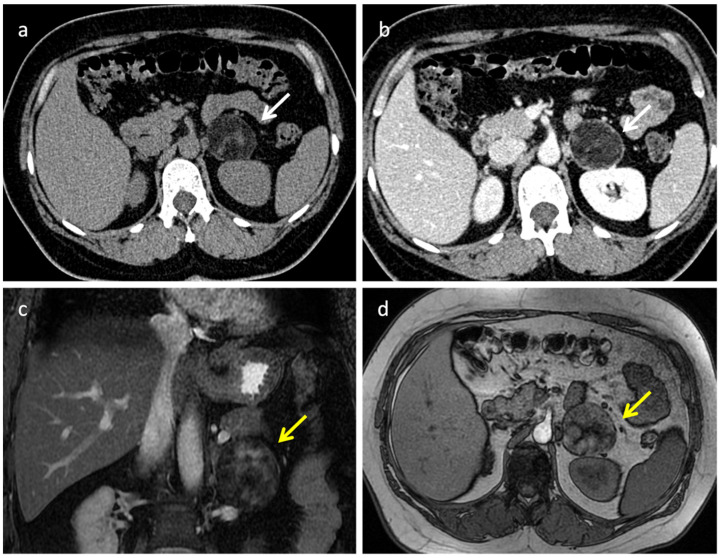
Adrenal myelolipoma in a 42-year-old woman. CT demonstrates a large mass within the left adrenal gland (white arrow in (a,b) and yellow arrows in (c,d)), characterized by well-defined borders and internal inhomogeneity. Axial unenhanced CT (a) shows attenuation values <−30 HU due to the presence of gross fat. On portal venous phase (b), the mass shows subtle enhancement due to its poor vascularization. Coronal T2 fat sat sequence (c) and axial T1-weighted out-phase image (d) demonstrate extracellular fat.
